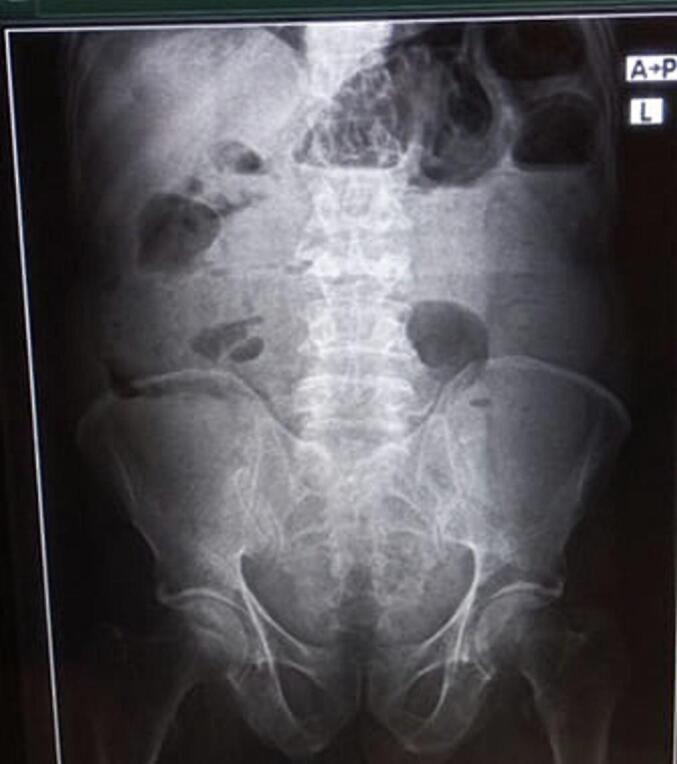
Plain abdominal X-ray of the patient suggesting small bowel obstruction.
